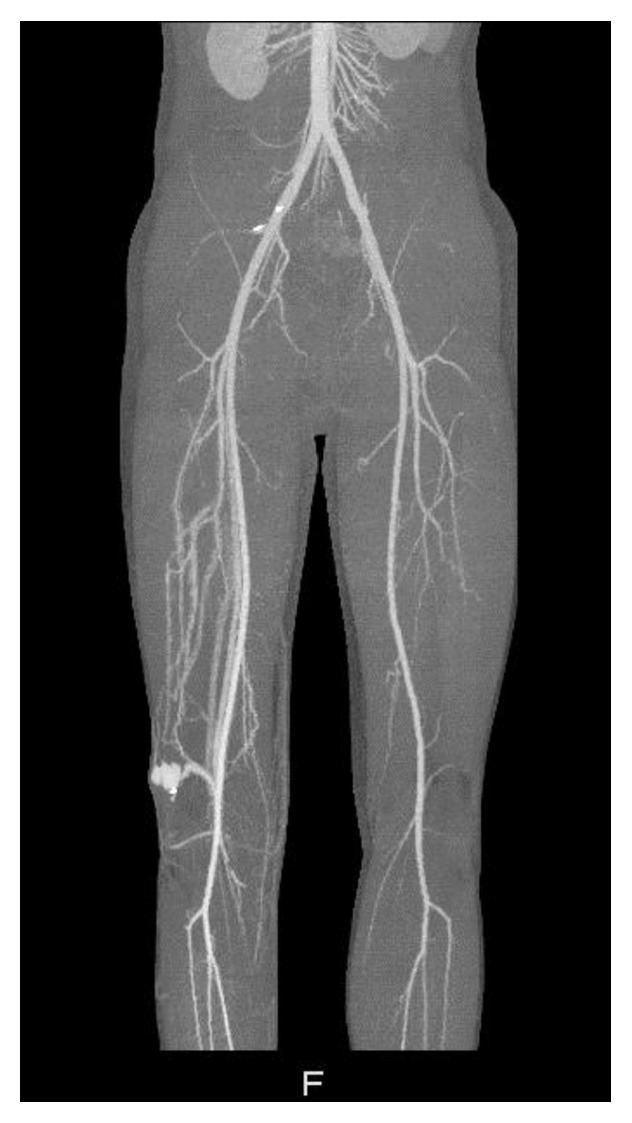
CT angiography showing the pseudoaneurysm and AV fistula lateral of the right knee.
